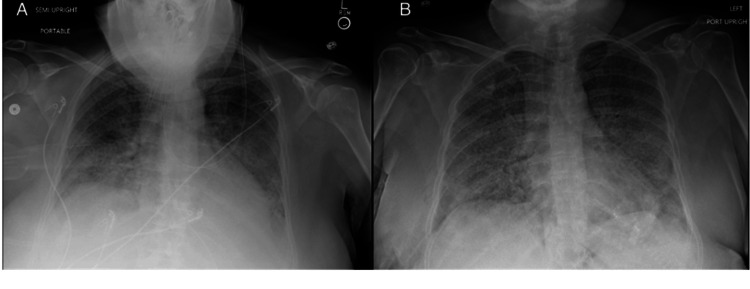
Chest X-rays showing severe diffuse lung infiltrates on hospital day 2 (A) and day 21 (B).
